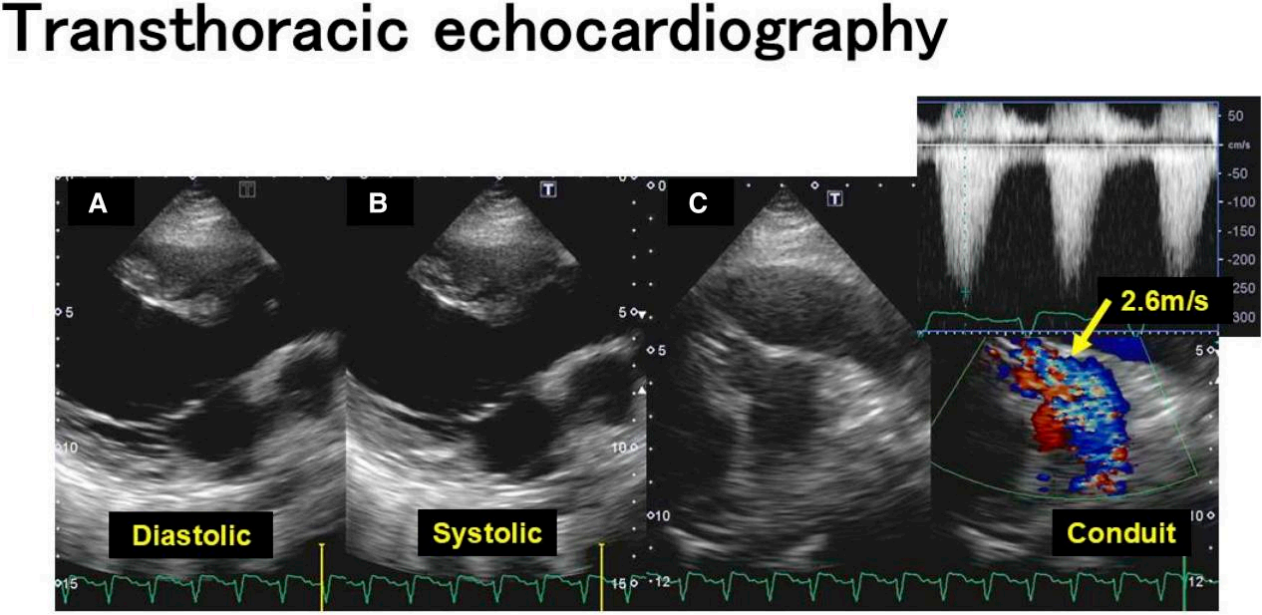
Transthoracic echocardiography at the admission to our hospital. (*A*, *B*) Parasternal long-axis view showing left ventricle at diastolic and systolic. Left ventricular ejection fraction (LVEF) 21%, left ventricular end-diastolic dimension 55 mm, and end-systolic dimension 49 mm. (*C*) Rastelli conduit stenosis which peak flow velocity was 2.6 m/s (indicated by the arrow).
